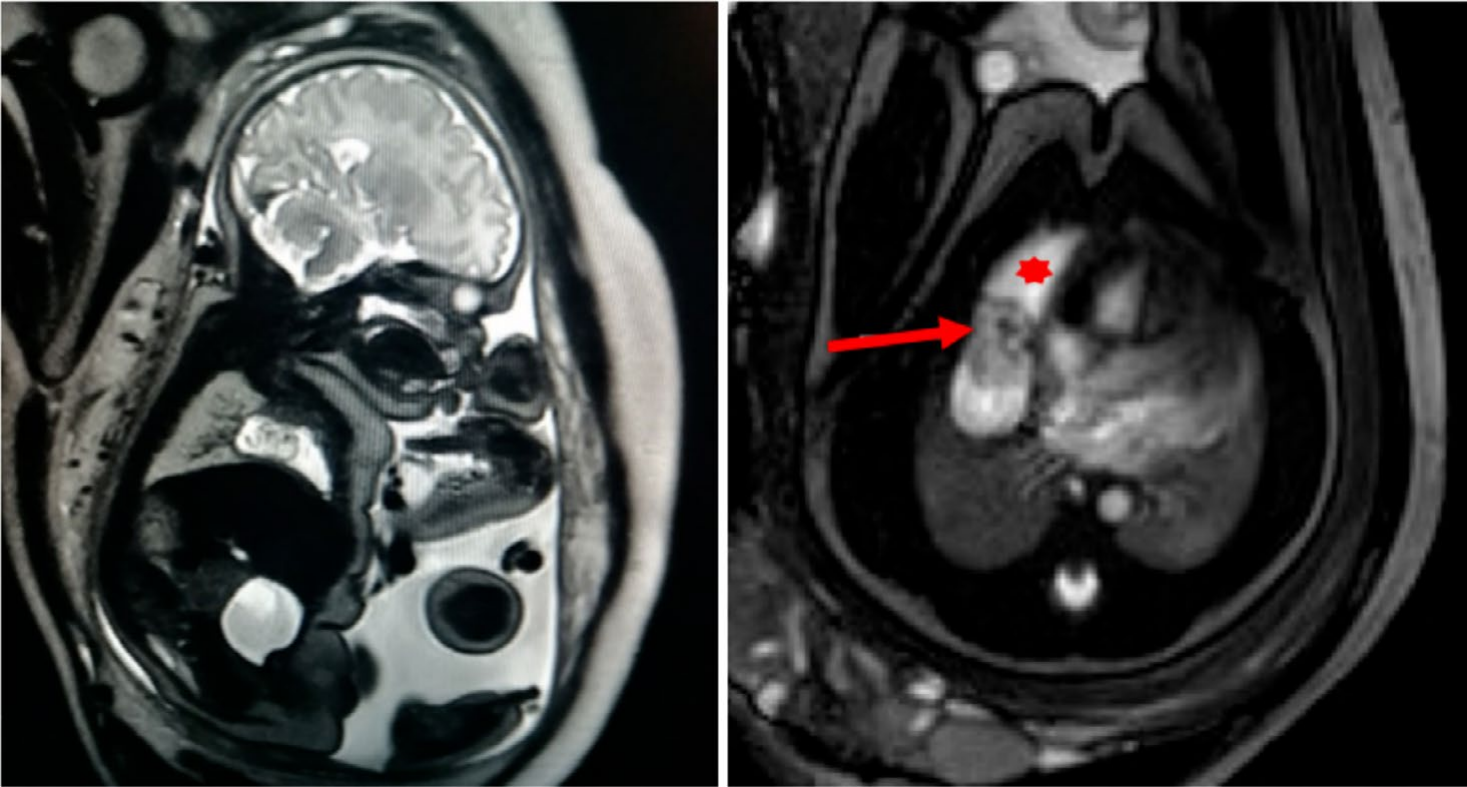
Foetal MRI. Axial balance turbo field echo (b‐TFE) image shows a well‐defined cystic lesion (asterisk) lying in the right anterior cardiophrenic angle, with an inhomogeneous, nodular, solid low‐signal lesion within it (arrow). No concurrent pericardial effusion is noted.
